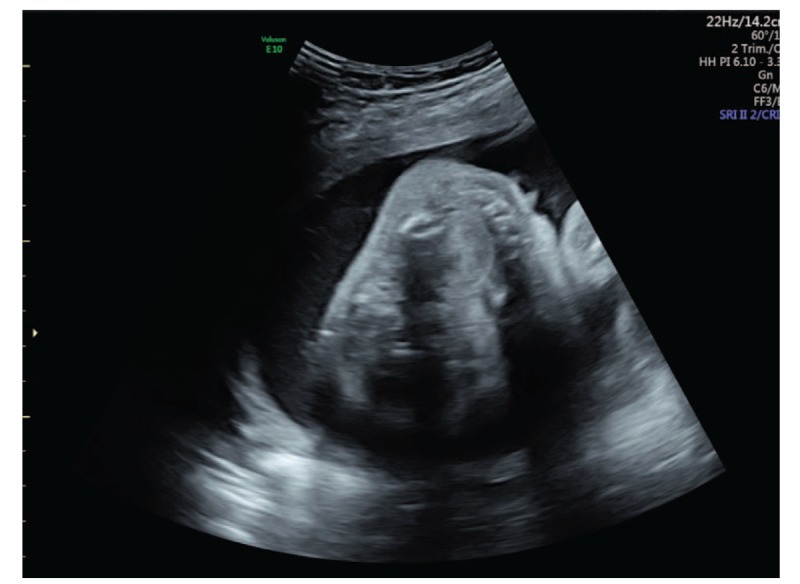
Two-dimensional ultrasound of the fetal tongue reached the anterior mandibular alveolar ridge.
